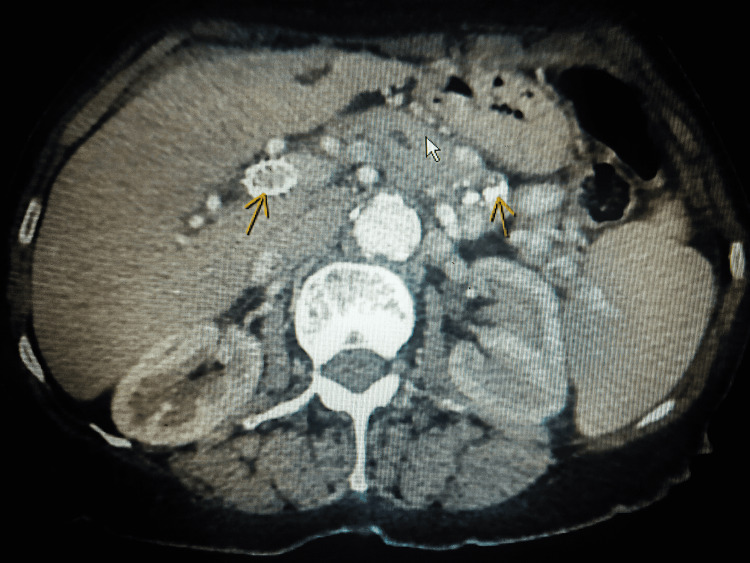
Contrast-enhanced computed tomography (CECT) of the abdomen showing patent TIPS (arrow on the left), dilated pancreatic duct (white arrow), areas of calcification in the pancreatic head (arrow on the right)
